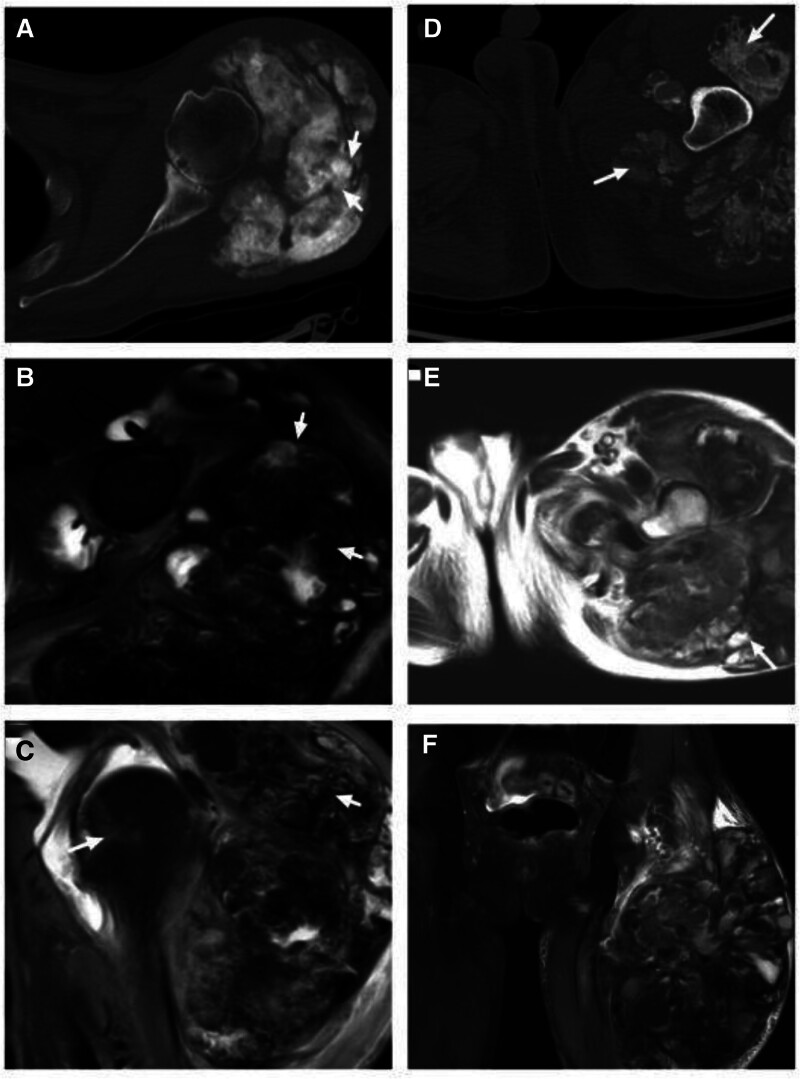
(A) CT showed thickening of soft tissue around the left shoulder joint, with clumpy lobed soft tissue shadows (white arrow). (B) MRI showed mixed signals around the shoulder joint, with cystic abnormalities mixed with low/high/slightly high T2 signals (white arrow) in the inner part. (C) Coronal MRI showing fluid accumulation in the left shoulder joint with bone marrow edema (white arrow). (D) CT showed mass mixed with high-density tissue around the left hip joint (white arrow). (E) MRI showed abnormal mixed signals in soft tissue masses around the left hip joint, partially sass-like (white arrow). (F) Abnormal cystic mixed signals were observed in coronal position. CT = computed tomography, MRI = magnetic resonance imaging.
